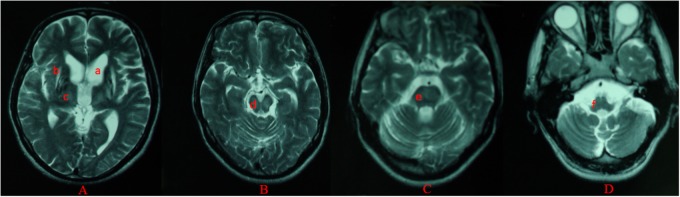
Different levels of brain MRI (T2WI) in a 33-year-old male (A–D). axial images show ventricular widening (a) and hyperintensity in putamen (b), thalamus (c), mesocerebrum (d), pons (e), and medulla (f).
